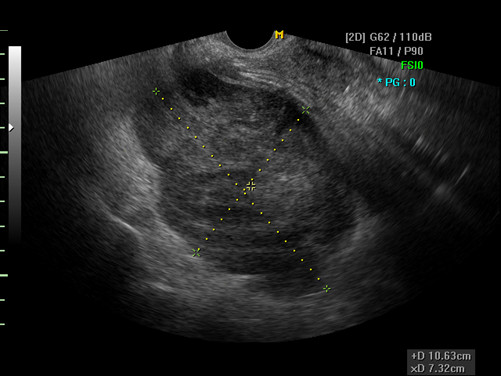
Transvaginal ultrasound showing mixed echogenic, solid mass, measuring 10.6 × 7.3 cm.
